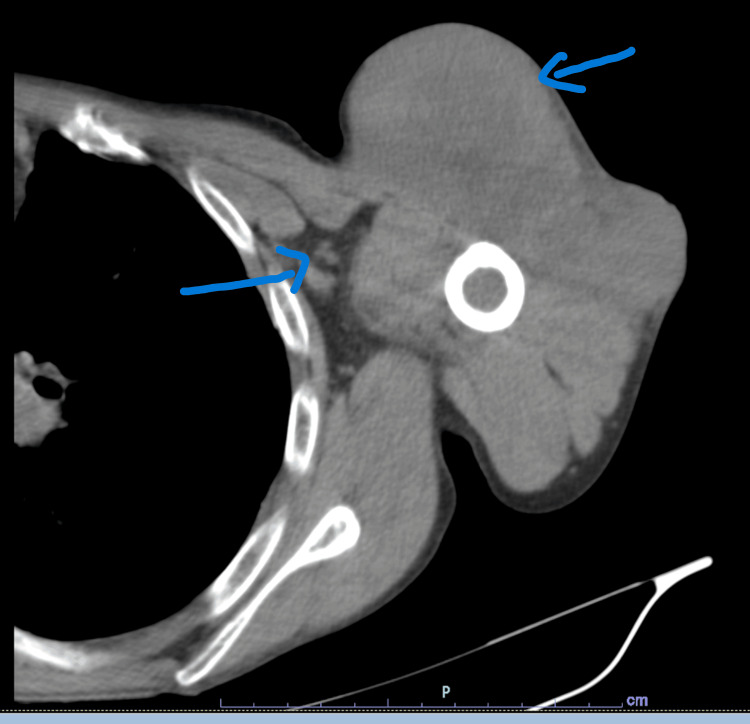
Axial CT image showing a necrotic lesion in the anterior deltoid muscle with peripheral enhancement.
